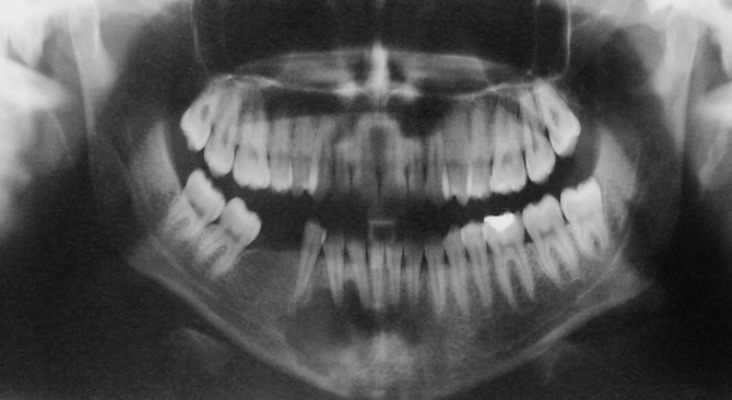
In panoramic radiography was revealed unilocular radiolucency in periapical area of anterior mandibular teeth.
